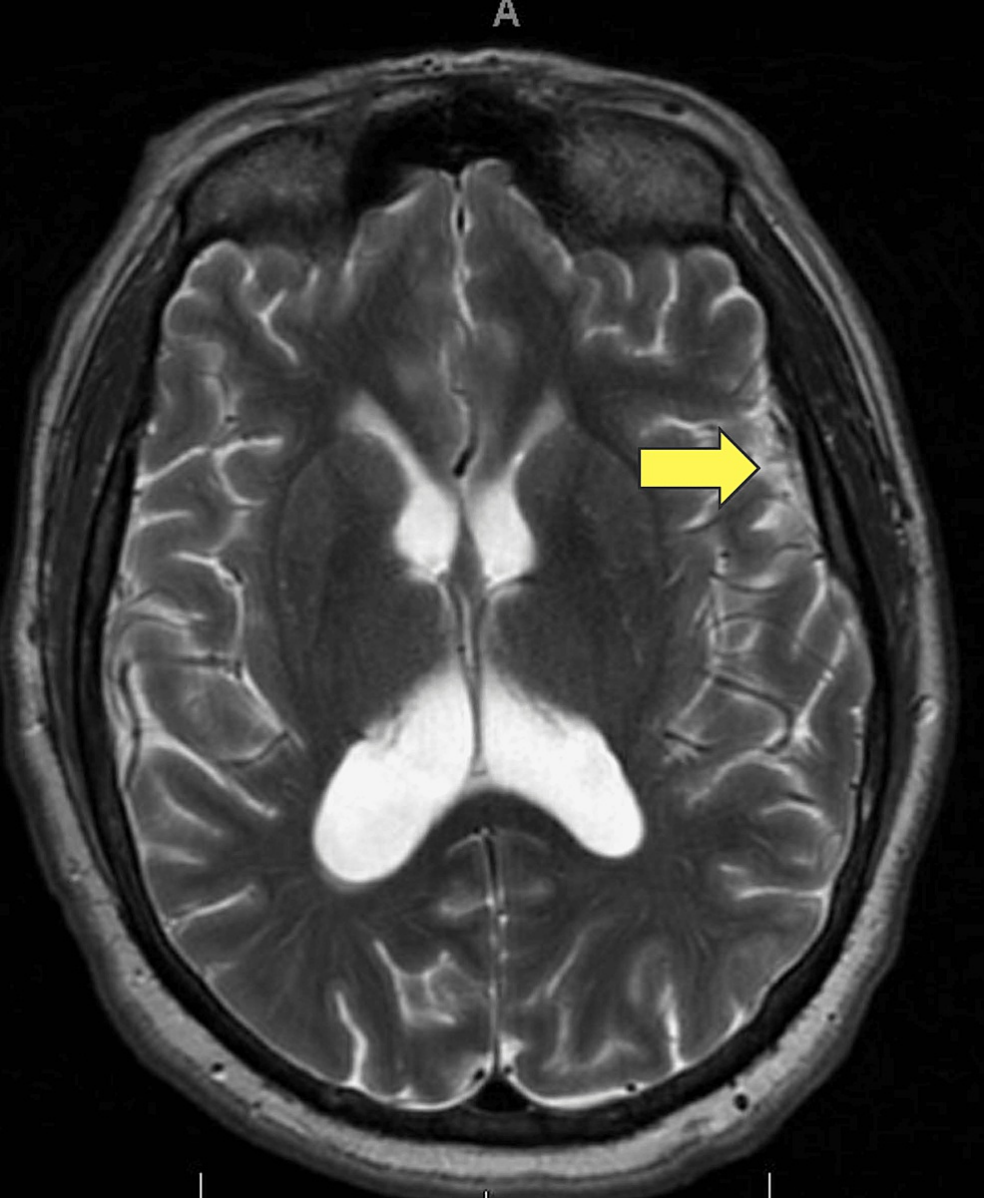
Brain MRI-seizure protocol without and with gadolinium showed an interval decrease of the left frontal region, which previously measured 6 mm in thickness, now measuring 4 mm.
